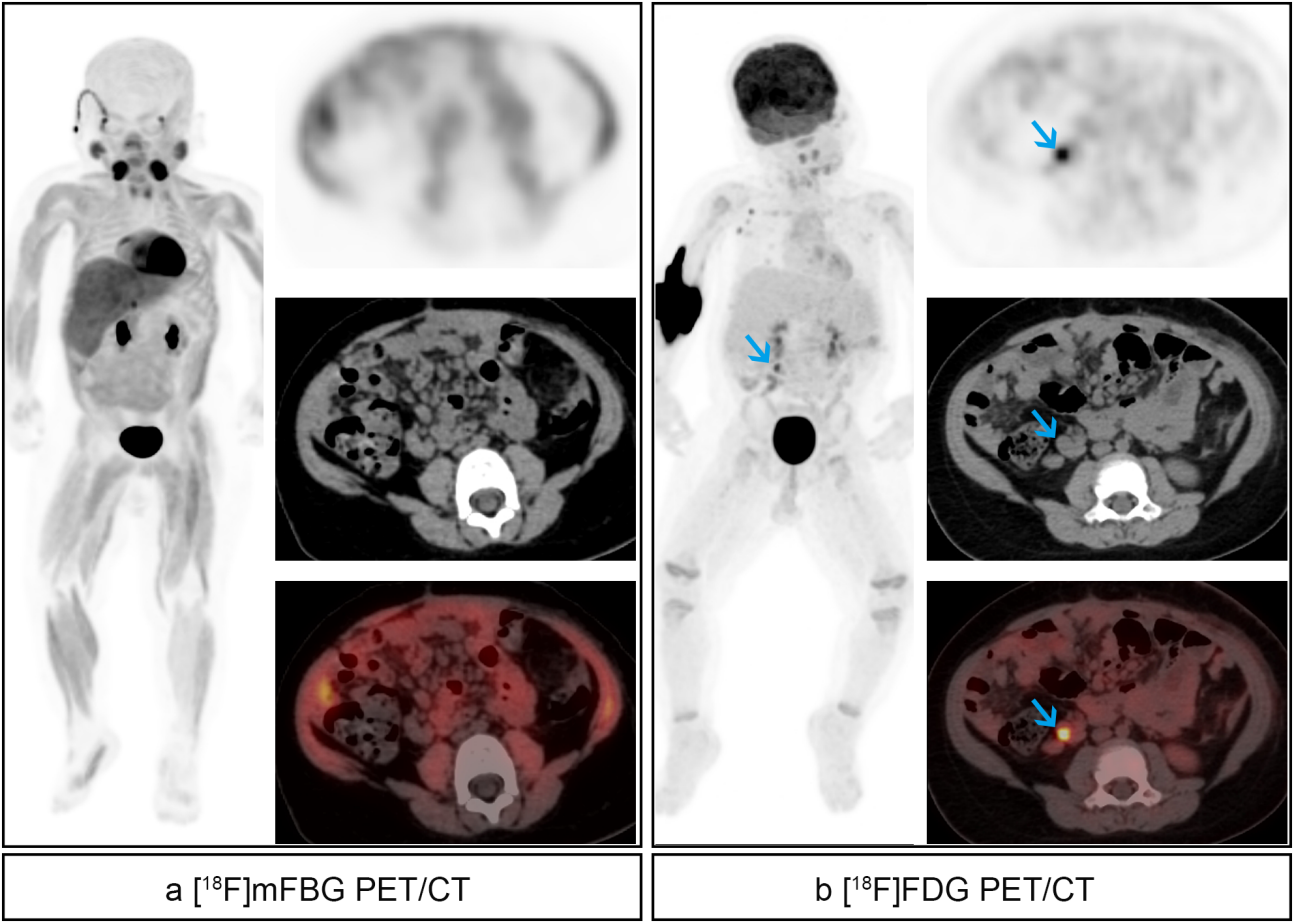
A 2-year-old boy suffered low-risk left retroperitoneal neuroblastoma and followed by 2 cycles of chemotherapy. Post-therapy images were acquired. (a) [^18^F]mFBG images. No residual activity was found. (b) [^18^F]FDG images. Abnormal activity (blue arrows) with SUVmax 5.0 and TBR 11.6 was found in the right retroperitoneal lymph node.
